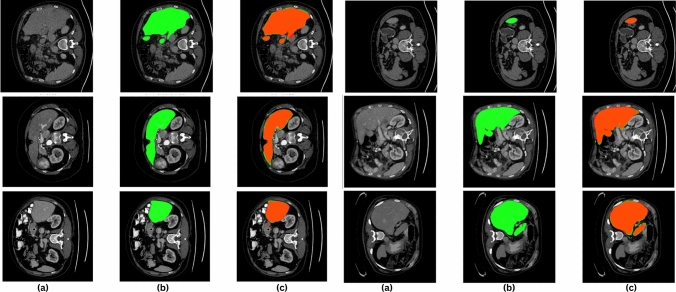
Qualitative Liver Segmentation Overlays Using SLIC-Former. (a) Original abdominal CT slices. (b) Ground-truth liver masks overlaid on the CT images (green). (c) SLIC-Former predicted liver masks overlaid on the CT images (red).
